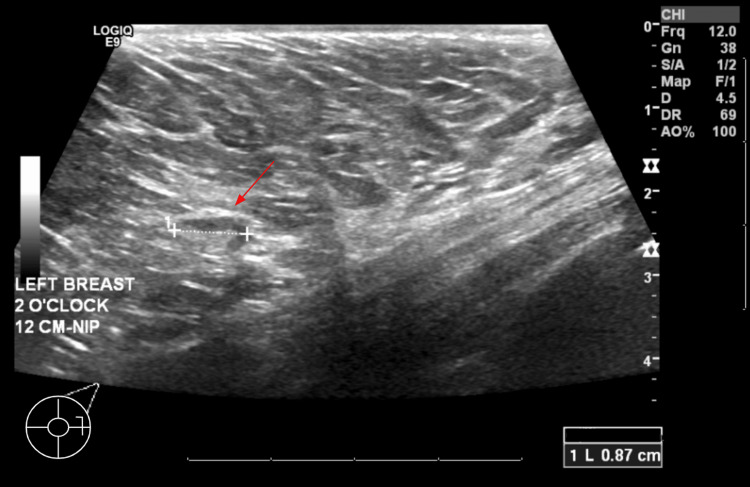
Targeted breast ultrasound. Initial ultrasound of the left breast at the 2 o'clock position, 12 cm from the nipple, demonstrates an intramammary lymph node measuring approximately 8.7 mm.
